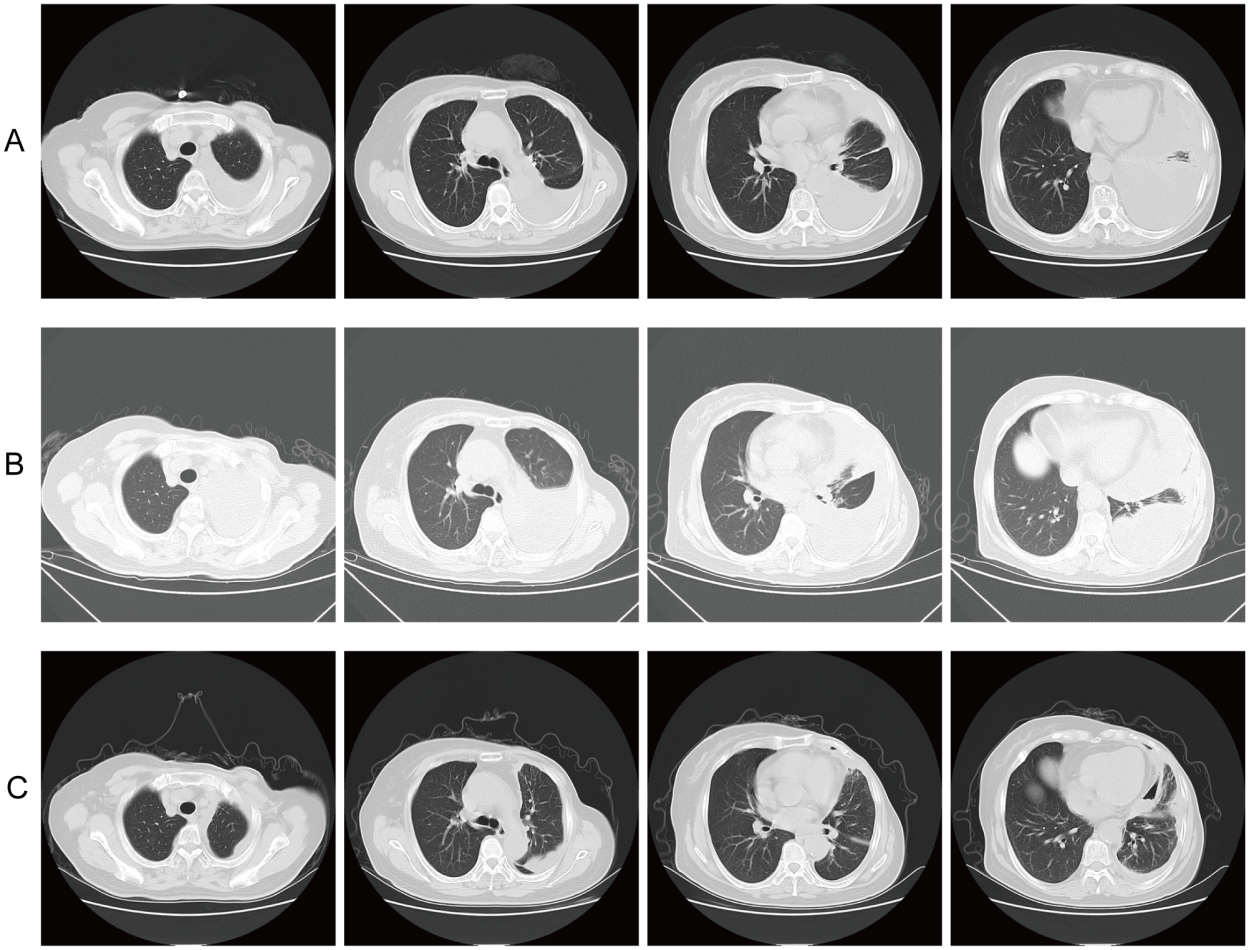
Serial CT imaging of left pleural effusion across three treatment phases at four anatomical levels. (A) Baseline (August 2024): Extensive pleural effusion before treatment with DS-8201 plus intrapleural cisplatin. (B) Post-cisplatin (October 2024): Increased pleural effusion after 3 cycles of DS-8201 plus intrapleural cisplatin. (C) Post-bevacizumab (November 2024 onward): Marked reduction of pleural effusion after switching to DS-8201 plus intrapleural bevacizumab.
